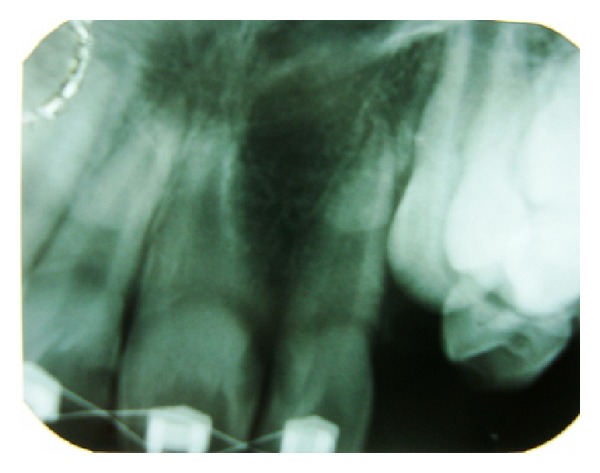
Intraoral periapical radiograph shows root parallelism of 21 and 22.
